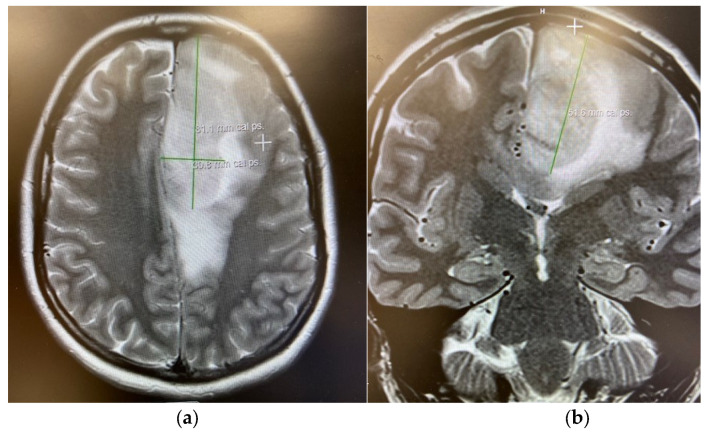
MRI images from clinical case. MRI brain demonstrates T2 hyperintense lesion and surrounding infiltration on an axial (a) and coronal (b) image on this axial T2 sequence.
